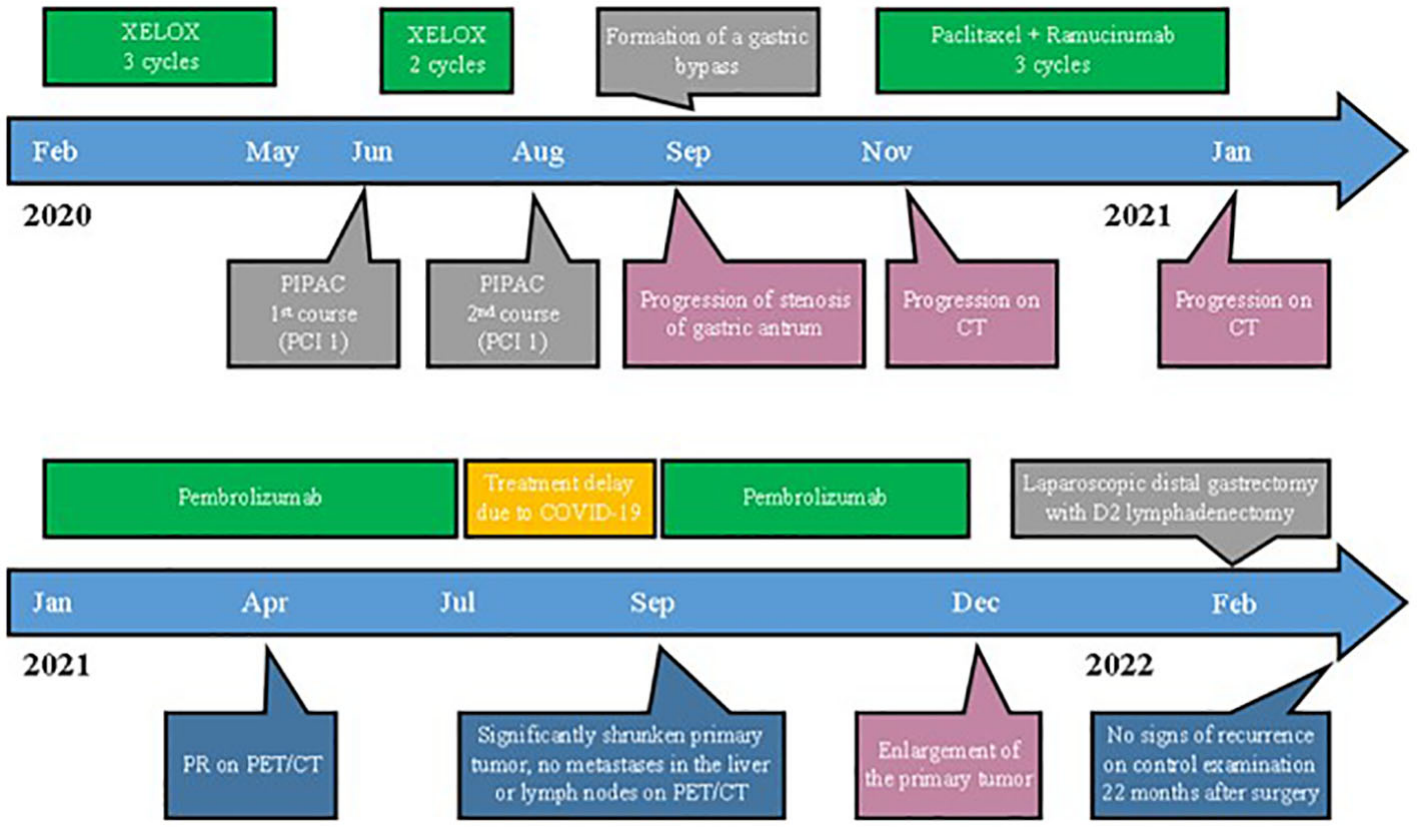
Timeline of the treatment and disease characteristics. PIPAC, pressurized intraperitoneal aerosol chemotherapy; PCI, peritoneal cancer index; CT, computed tomography.
